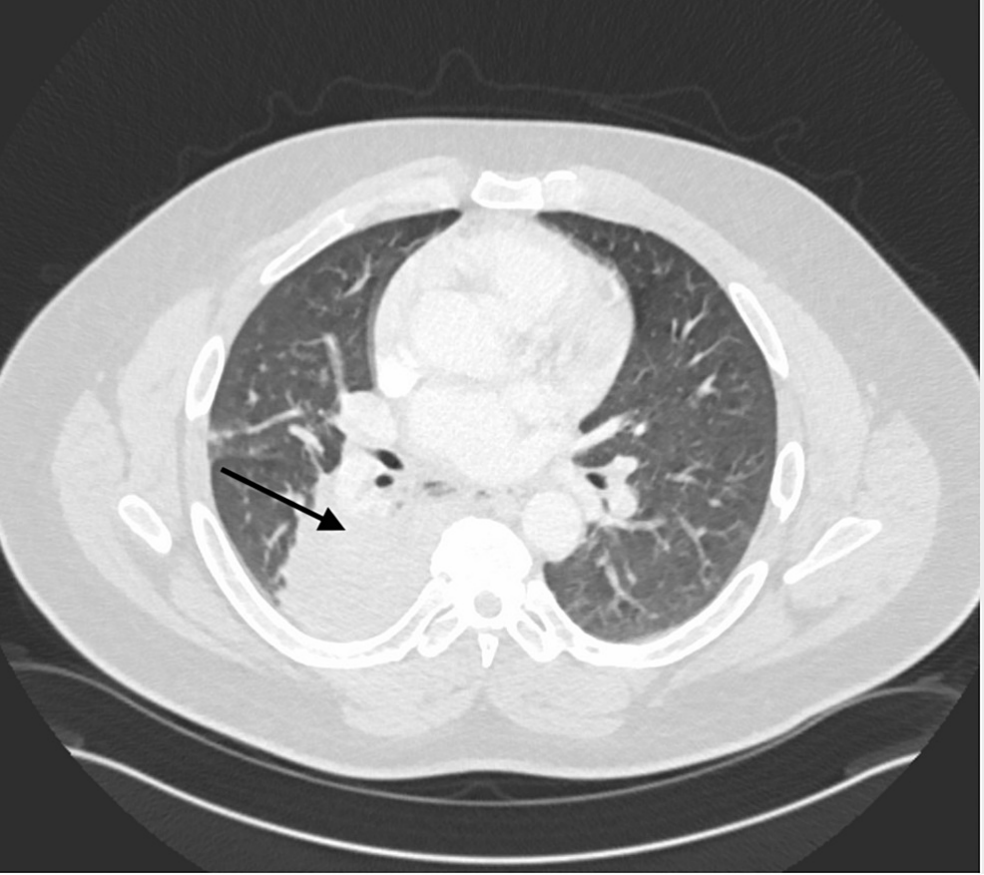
Chest CT demonstrating airspace consolidation of the right middle and lower lobe suggestive of pneumonia CT: computed tomography
